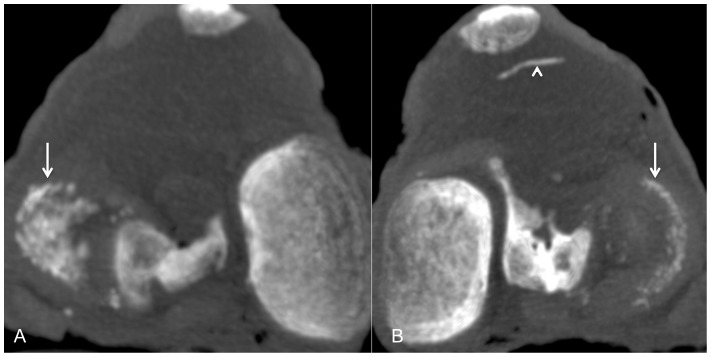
Computed tomographic scans of two different knees on transverse sections (A–B) in Maximum Intensity Projection (MIP, thick section of 3 mm) demonstrating diffuse calcifications within the three meniscal segments (white arrows). Vascular calcifications were observed on the transverse section B (arrow head).
